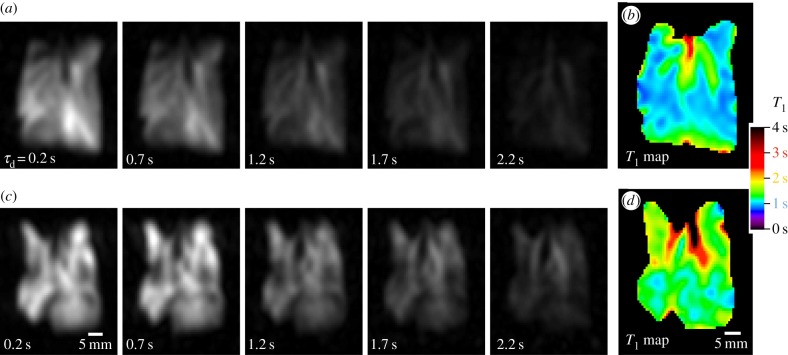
Series of hp ^83^Kr MR images with resultant *T*_1_ maps in control and PPE-treated lungs. Each image was acquired using a new delivery of hp ^83^Kr. (*a*) VFA FLASH MRI with no slice selection in control lung CL.2, using a variable relaxation delay, *τ*_d_, ranging from 0.2 to 2.2 s between hp gas inhalation and acquisition as indicated in the figure. Note that the major airways are less affected than the lung parenchyma by increasing *τ*_d_ values. (*b*) The resultant SQUARE *T*_1_ map for the control lung displays longer *T*_1_ values (green) for the major airways and shorter values for the alveolar region (blue). (*c*) VFA FLASH MRI as in (*a*) for the PPE-treated lung EL.1. The lung exhibits heterogeneity in ventilation that is likely caused by the PPE treatment but is not a clear indicator for emphysema. (*d*) The resultant SQUARE *T*_1_ map of the PPE-treated lung displays prolonged relaxation times (green) in the alveolar area compared with the control SQUARE *T*_1_ map above.
